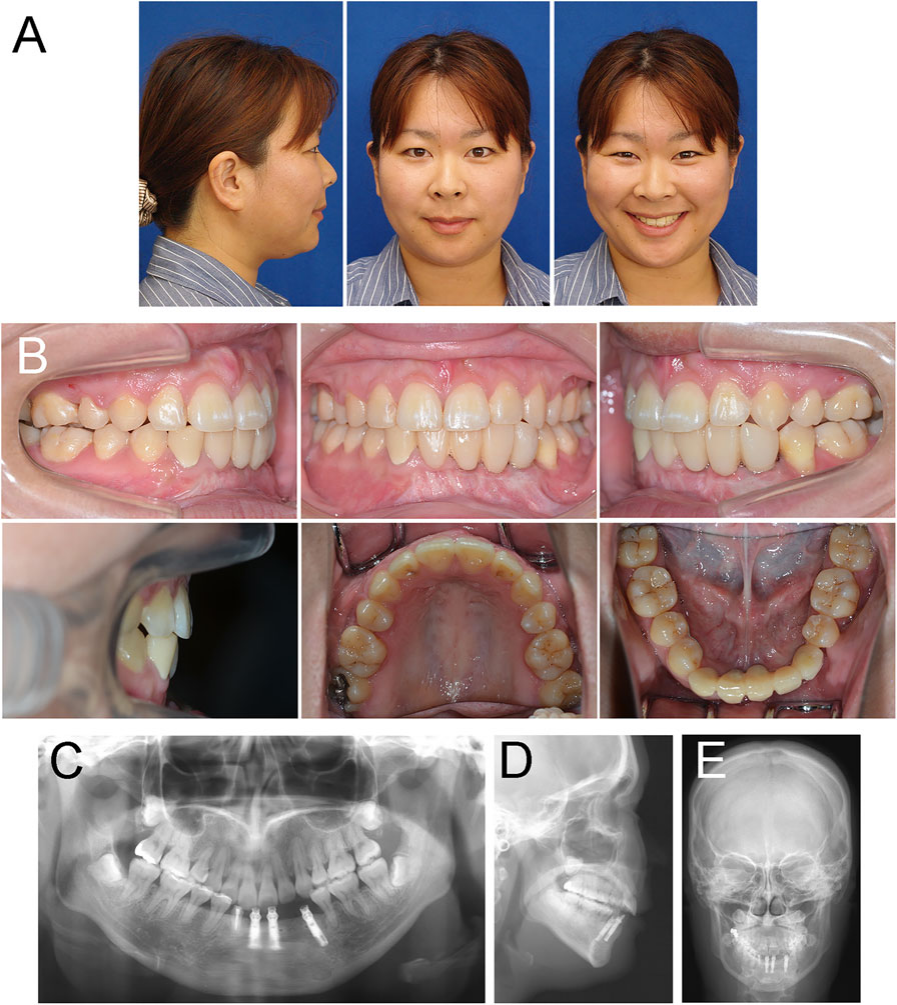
The post-treatment recordings. a Extraoral photographs. b Intraoral photographs. d A panoramic radiograph. c A lateral cephalogram. e A posteroanterior cephalogram
